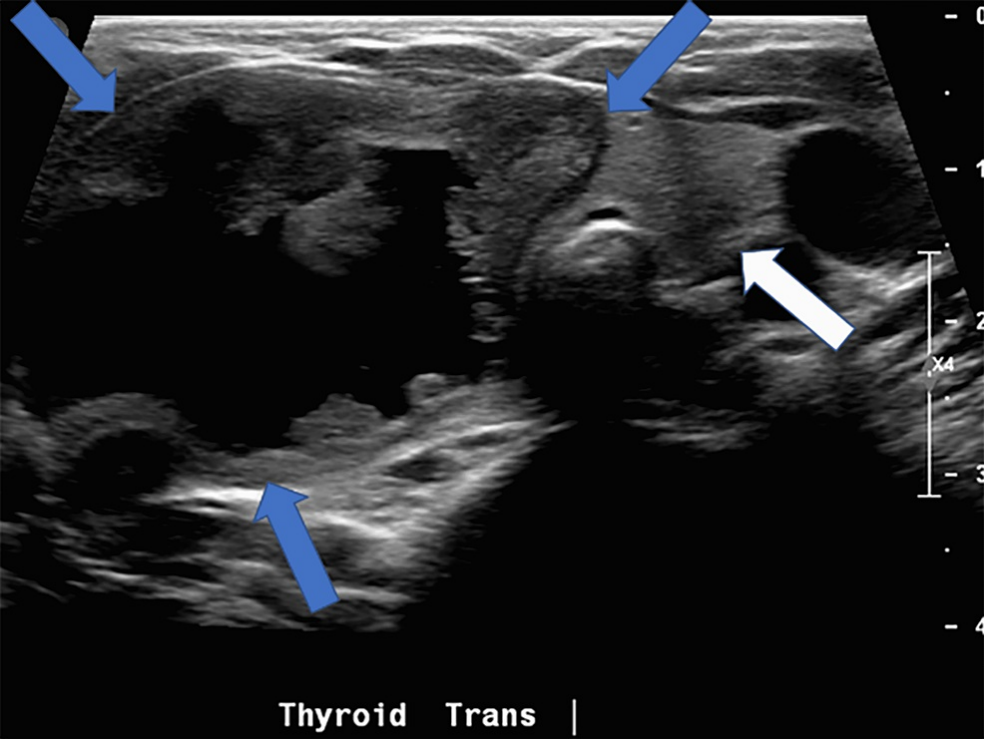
Transverse ultrasound image of the thyroid gland demonstrates a circumscribed, solid mass containing a hypoechoic, cystic component with irregular walls confined to the right lobe (blue arrows). The left lobe of the thyroid gland appears normal (white arrow). Image courtesy: This image was provided by Dr. Laura L. Hayes from the radiology department at Nemours Children’s Health System.
